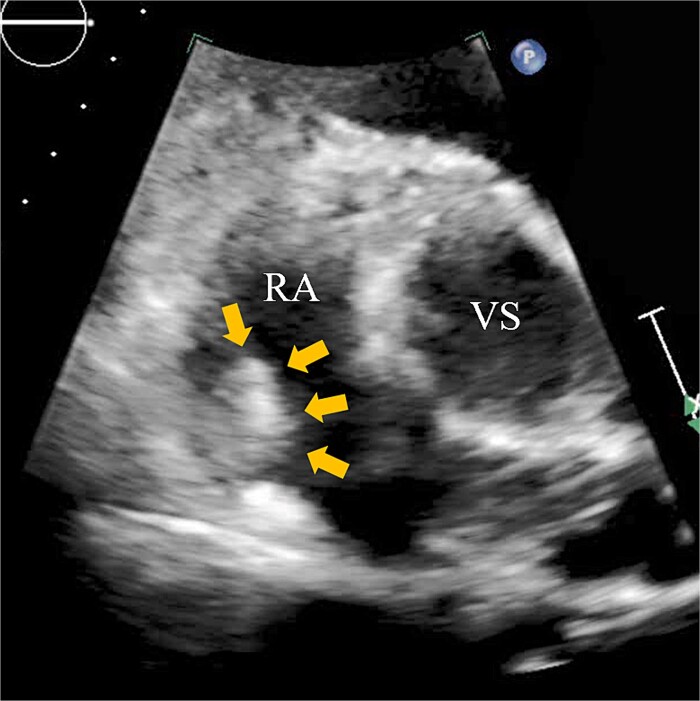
Transthoracic echocardiography showing a right atrial mass measuring 17 × 19 mm with slight mobility (arrows). VS, Valsalva sinus.
